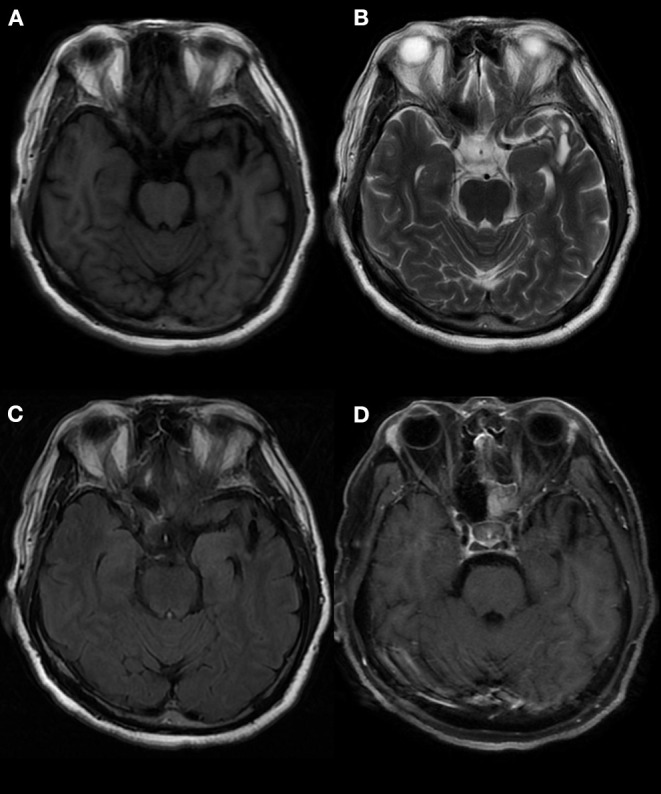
Abnormal signals in the left temporal lobe, as indicated by brain MRI. MRI showed an oval mixed signal shadow in the left temporal cortex, a low signal in the FLAIR scan, a wide left fissure cistern, and no enhancement in the contrast-enhanced scan. MRI: magnetic resonance imaging. (A) T1 (T1-weighted imaging). (B) T2 (T2-weighted imaging). (C) FLAIR (fluid-attenuated inversion recovery). (D) T1C (MRI enhanced scan).
